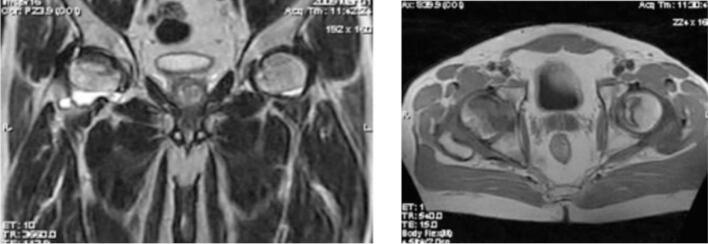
Pre-operative MRI coronal and axial view (Low signal intensity at bilateral femoral head) for patient II.
